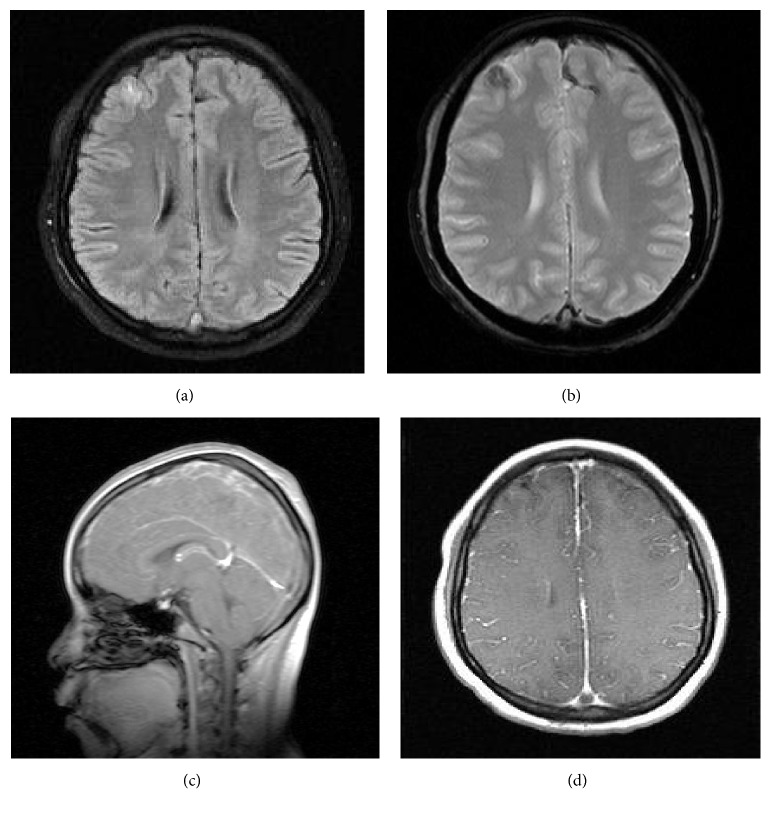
Initial MRI brain of our patient with and without contrast showed right frontal hyperintensity on FLAIR (a) with corresponding area on gradient echo (b), suggestive of a small intraparenchymal hemorrhage. GRE also showed decreased signal of two frontal cortical veins (b). Sagittal T1 imaging (c) revealed heterogeneous signal of the superior sagittal sinus. Postcontrast images were remarkable for a filling defect with direct visualization of the thrombus (d) in the superior sagittal sinus.
